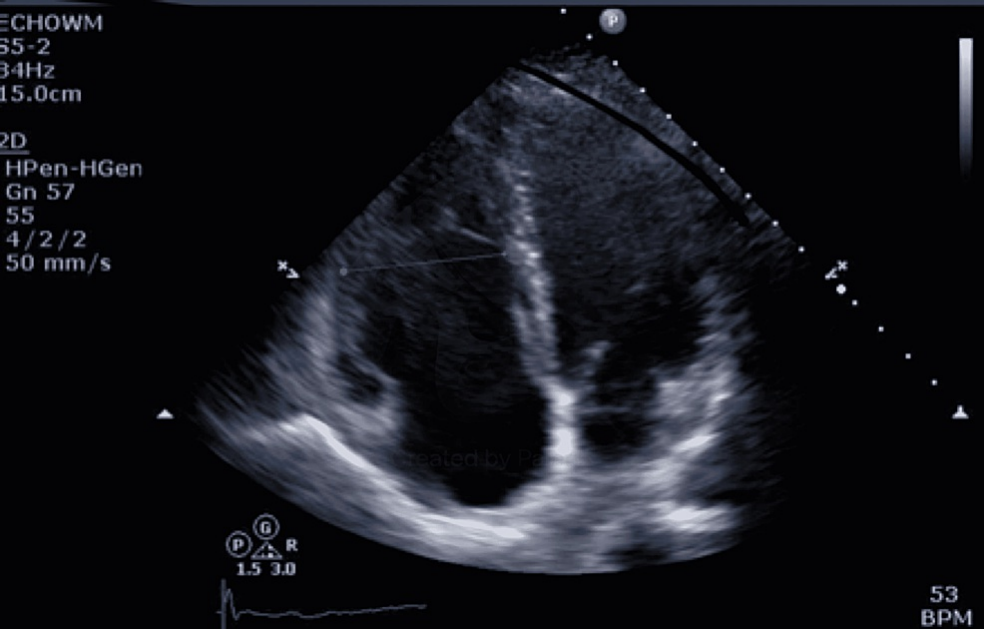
Echocardiogram showing mild dilatation of the right ventricle with a right ventricular systolic pressure of 28 mmHg
